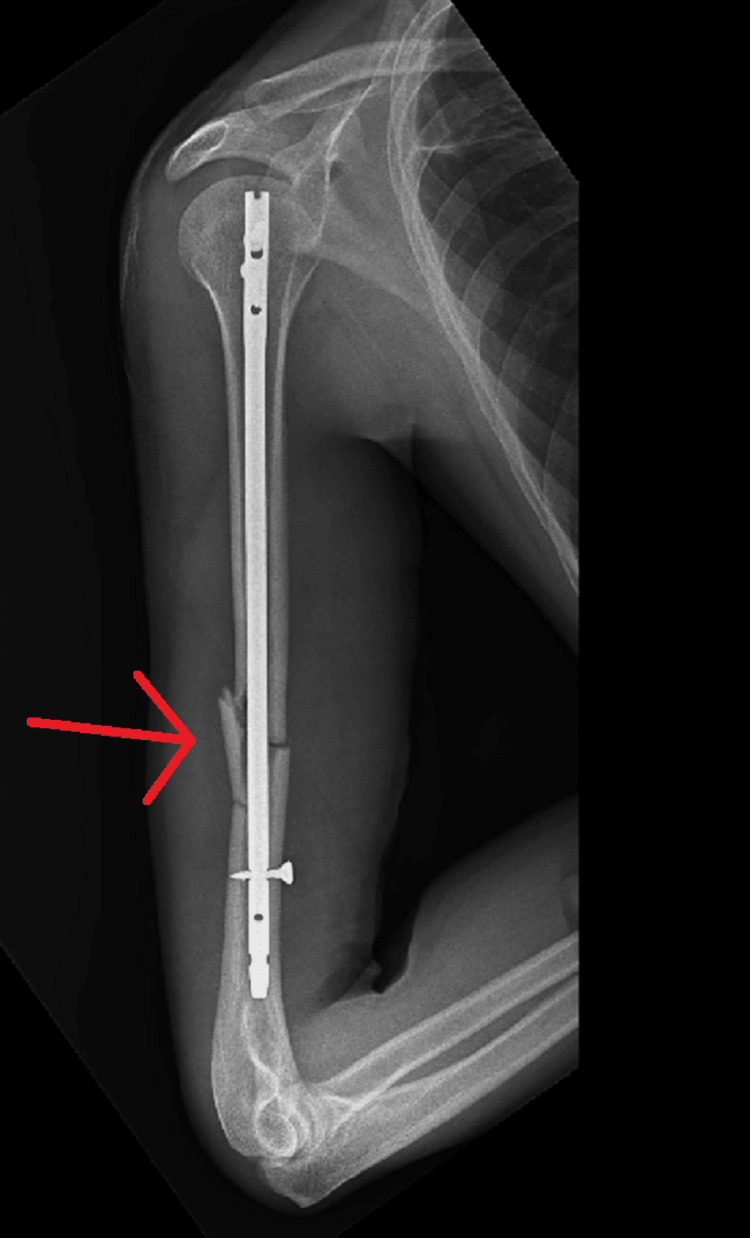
Lateral radiograph showing CRIF with intramedullary nailing for mid-shaft humerus fracture on the right side CRIF - closed reduction and internal fixation
